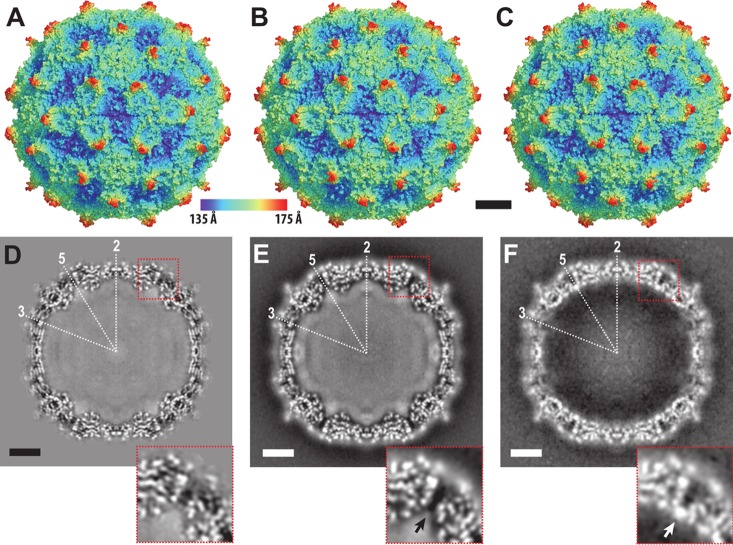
Structures of native IAPV virions and full and empty particles heated to 63°C. An X-ray structure of a native virion of IAPV (A) and cryo-EM structures of full virions heated to 63°C (B) and of empty particles prepared by heating virions to 63°C (C) are shown. The solvent-accessible surfaces are rainbow colored based on their distances from the particle center. Central slices of electron density maps of native virions (D), full virions heated to 63°C (E), and empty particles heated to 63°C (F) are shown in the bottom row. White areas indicate areas with high electron density values. The positions of selected icosahedral symmetry axes are labeled. The insets in panels D to F show details of electron density distributions near the 5-fold axes. The white arrow in the inset of panel F indicates a position of high electron density formed by putative ions. A corresponding density is not present in the virion structure, as indicated by a black arrow in the inset of panel E. Bars, 50 Å.
